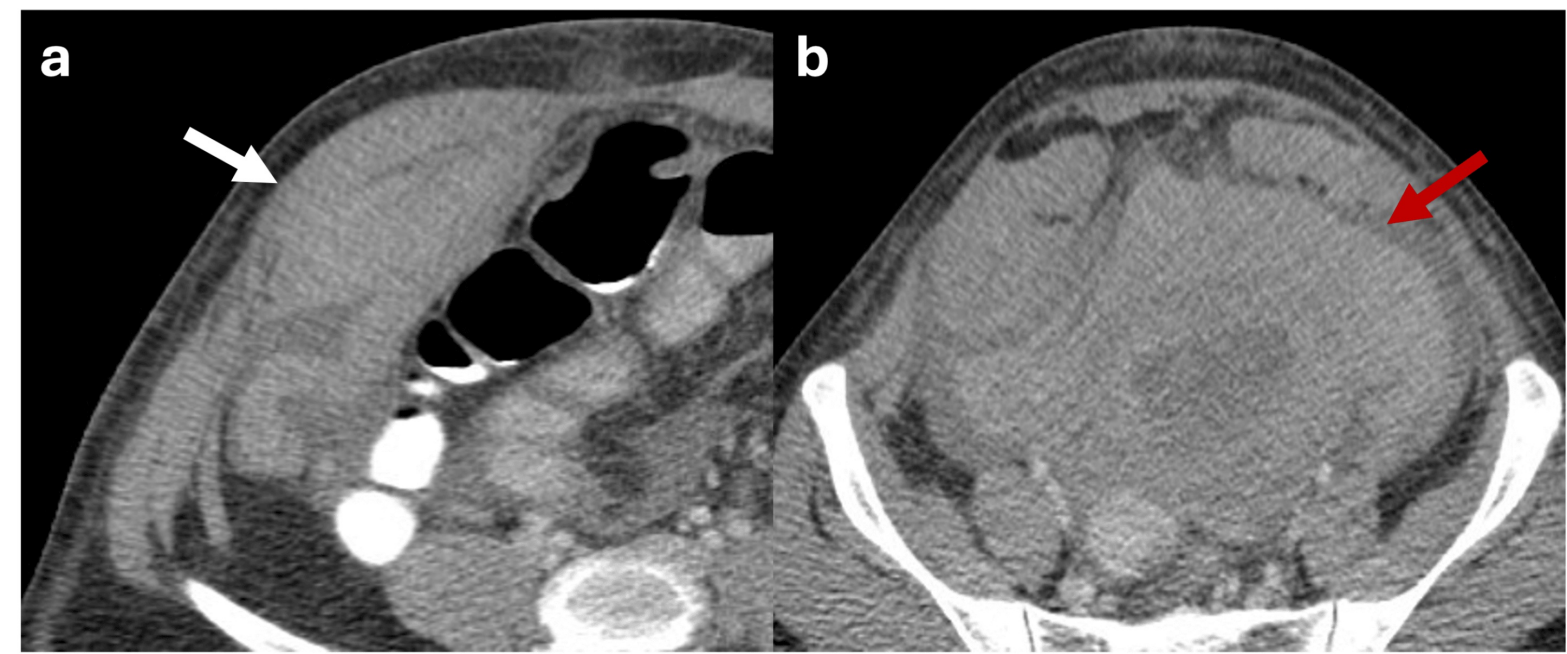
Axial abdominal CT scan showing a rectus abdominis muscle hematoma causing hemoperitoneum (a, b) Axial CT scan shows a hyperdense, non-enhancing lenticular collection within the right rectus abdominis muscle, corresponding to a hematoma (white arrow), notice that the hematoma extends below the arcuate line, rupturing into the abdominal cavity, causing hemoperitoneum (red arrow). The figure was created by the authors.
